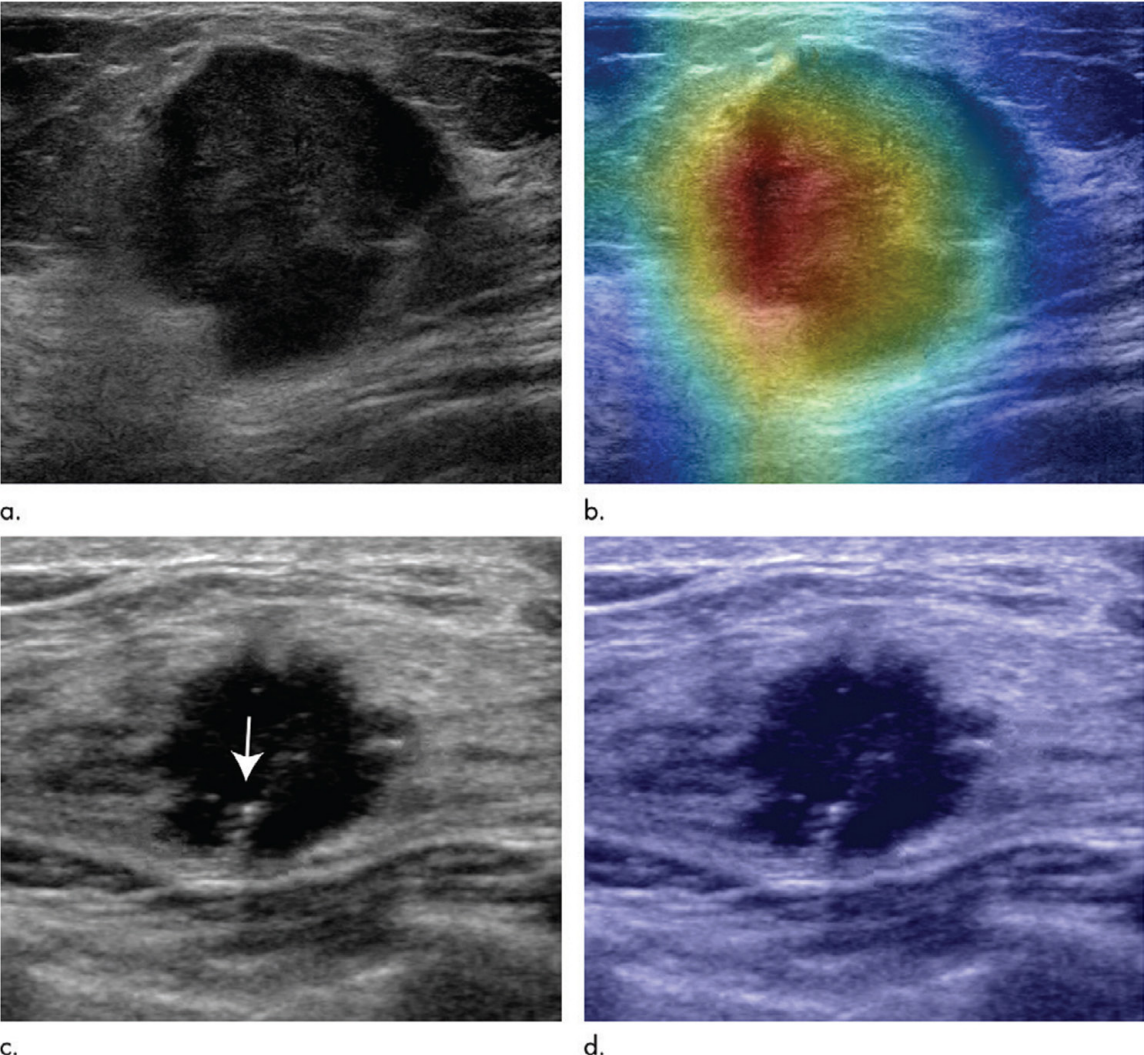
2D visualization of primary breast cancer in ultrasound images, and DL-aided prediction of clinically positive and negative lymph node metastasis.^
